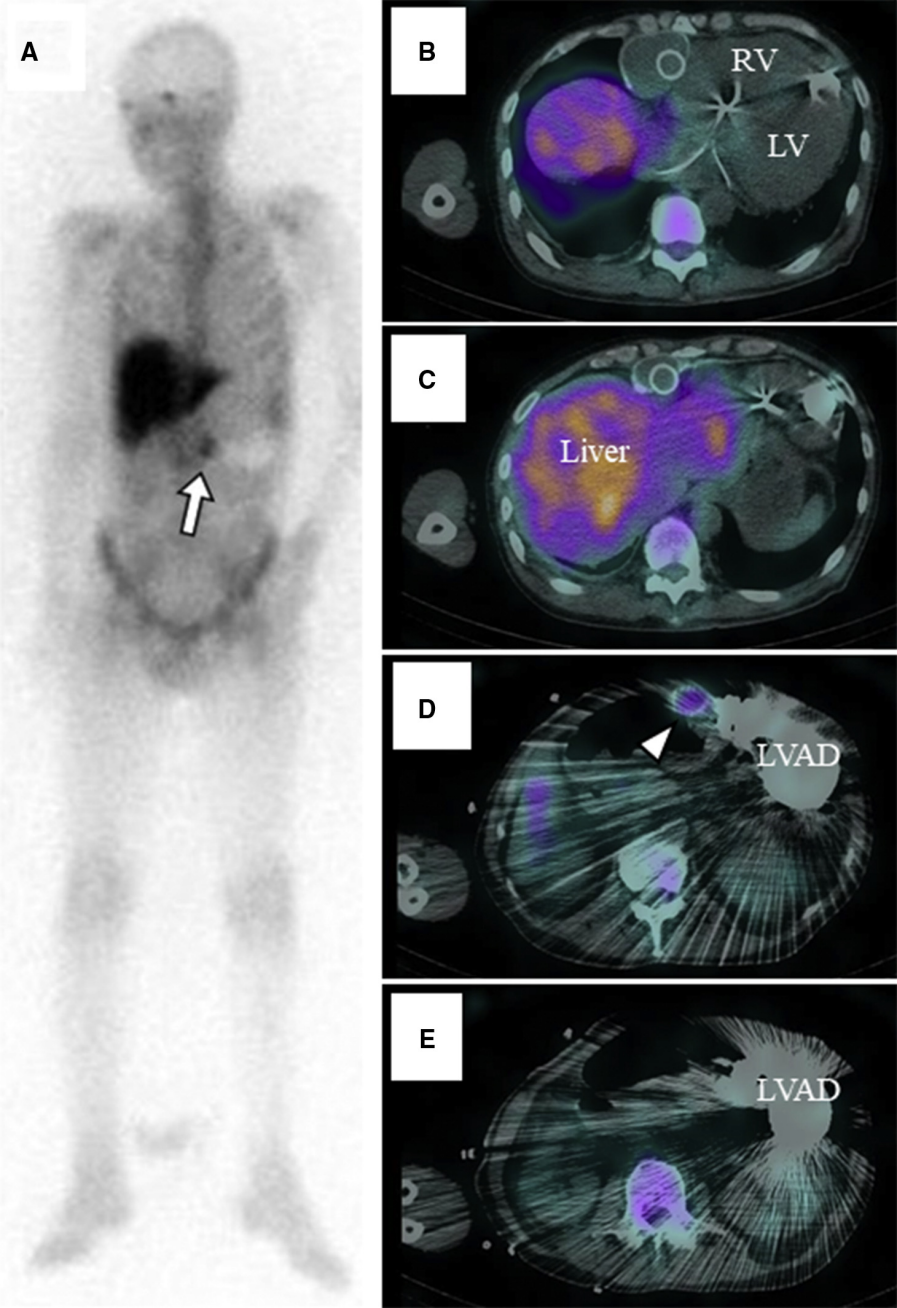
A, Gallium scintigraphy showed focal accumulation on the right side of the LVAD pump (*white arrow*). B-E, The site in which Ga-SPECT/CT accumulated was coincident with the outflow graft (*white arrowhead*). In addition, we could not find any uptake at the LVAD pump, pump pocket, driveline, and outside of outflow graft. *RV*, Right ventricle; *LV*, left ventricle; *LVAD*, left ventricular assist device.
